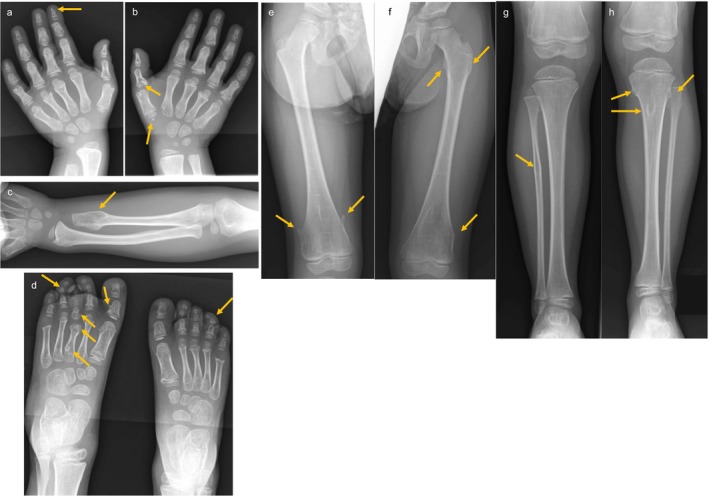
Radiographs of proband. (a,b) Multiple bilateral metacarpal and phalangeal osteochondromas. (c) Sessile protrusion on left ulna. (d) Multiple bilateral metatarsal outgrowths. (e) Pedunculated protrusions on distal left femur. (f) Outgrowths on proximal and distal right femur. (g) Suspected osteochondroma on proximal right fibula. (h) Outgrowths on left proximal tibia and fibula
